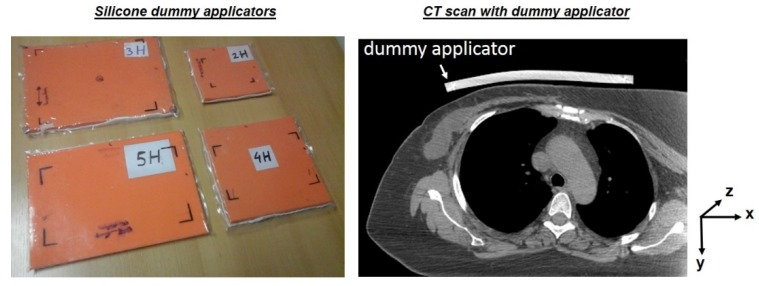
Photograph of four sizes of silicone dummy applicators (left) and a CT scan of a patient with a dummy applicator (right). As for the real antennas (Figure 1), the effective field size of the antenna is also marked on the dummy applicators.
